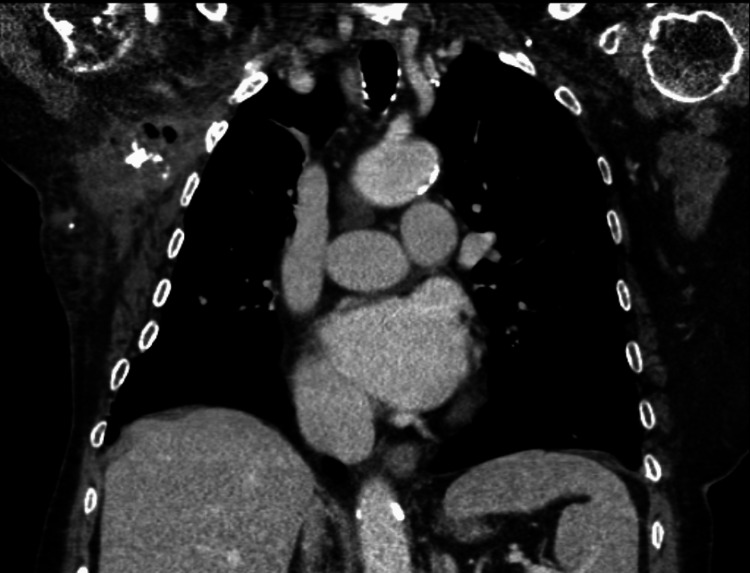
Coronal contrast-enhanced CT Coronal contrast-enhanced computed tomography showing extensive soft-tissue oedema and fat stranding of the right axilla with contiguous extension to the lower cervical and upper thoracic regions.
